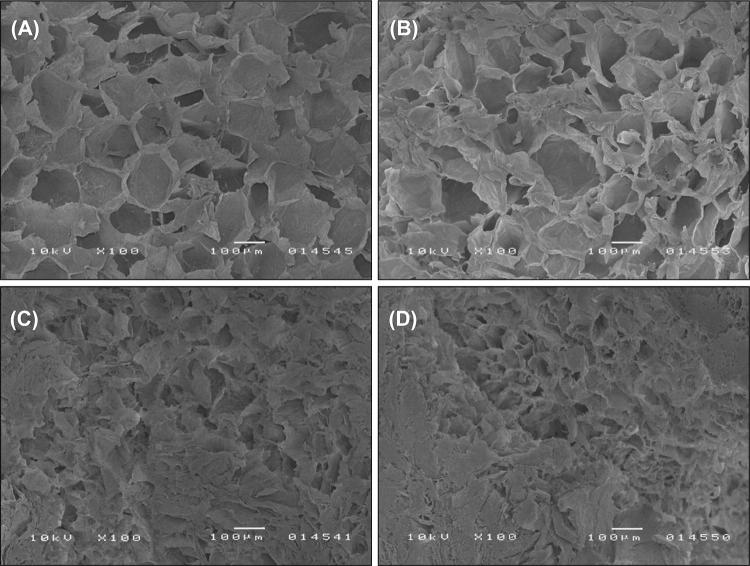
The microstructure of: (A) raw (untreated), (B) ultrasound-treated, (C) frozen/thawed, (D) frozen/thawed and ultrasound-treated blueberries
